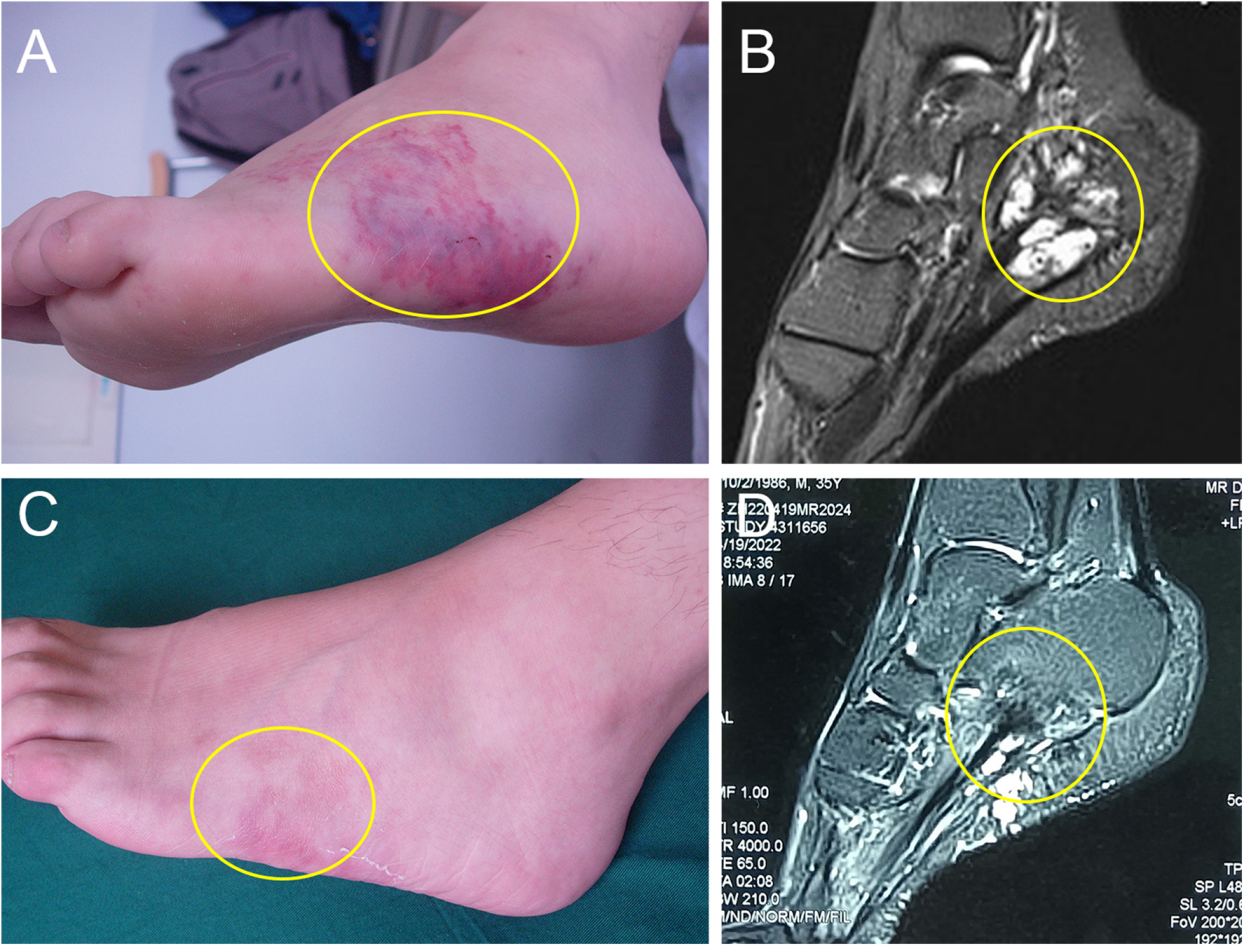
Diffuse infiltrative type IMVM in the left foot. (A) The immediate postoperative appearance. The local skin showed extensively damaged. (B) The MRI before treatment, which indicated that the lesions were located in the plantaris muscle. (C) The appearance after 4 weeks of treatment. The local skin was intact with no scarring. (D) The MRI after three times of treatment. The yellow ellipse indicated the lesion. The copyrights of the figures were held by corresponding author.
